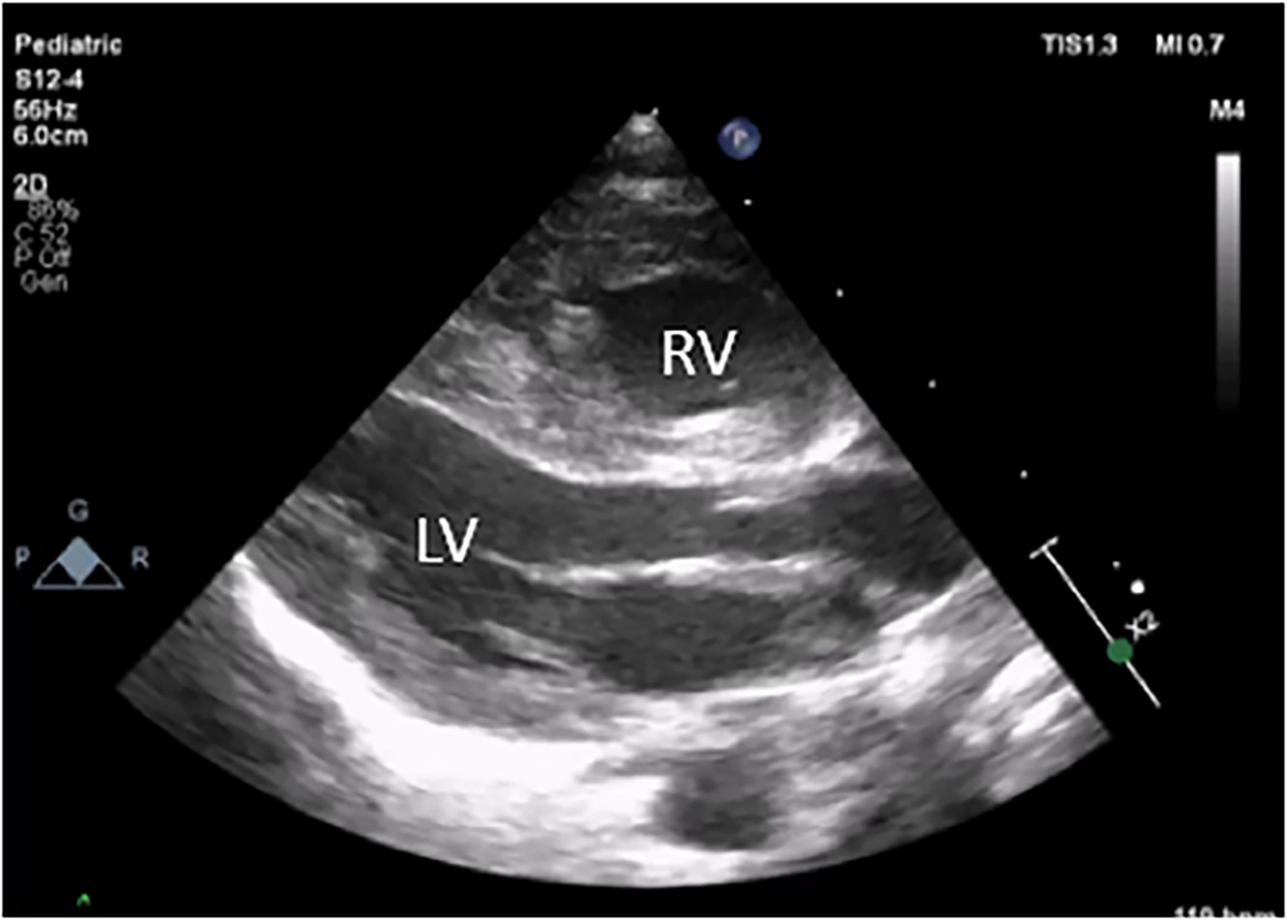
Echocardiogram parasternal long axis view demonstrating severe right ventricular hypertrophy (RVH). RV, right ventricle; LV, left ventricle.
